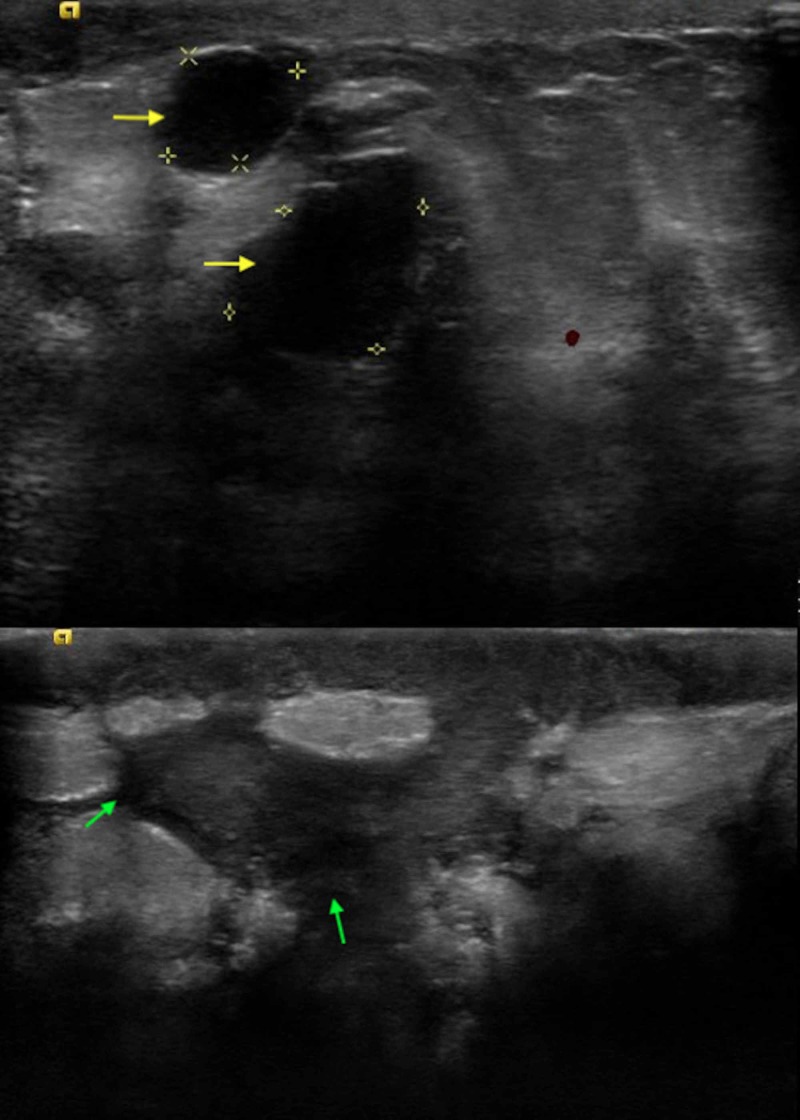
Breast ultrasound depicting multiple complicated cystic lesions (yellow and green arrows).
